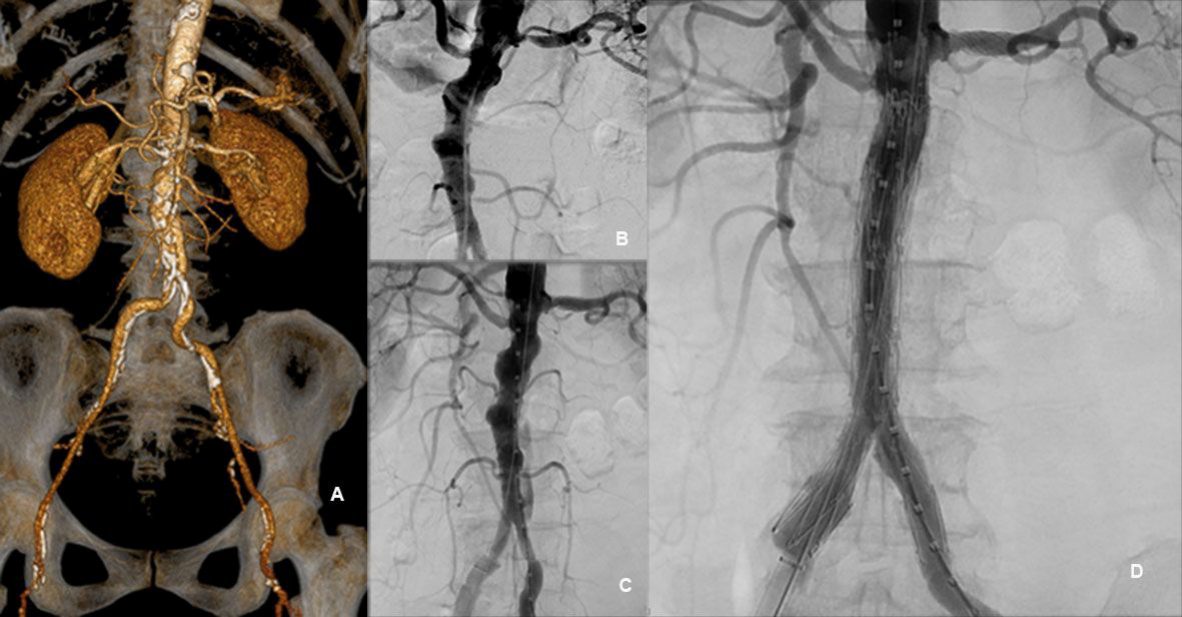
Example of “shaggy” aorta treated with main unibody bifurcated stent graft and proximal extension devices. (A) Pre-treatment Angio-CT image; (B) Intraoperative angiography; (C) Angiography after left renal artery stenting; (D) Post-treatment angiographic appearance.
